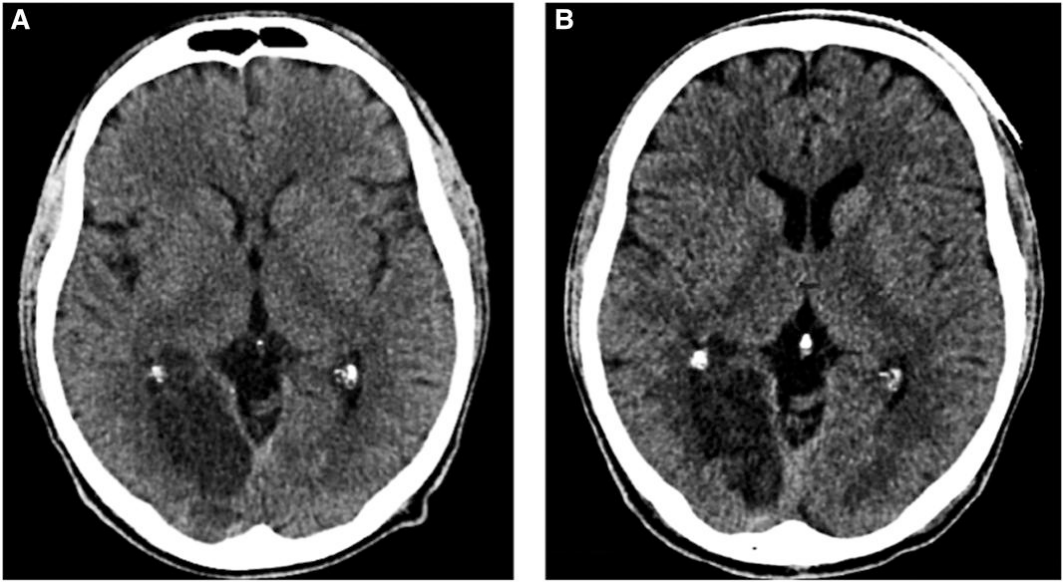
Brain CT scan without contrast performed in the emergency department (*A*) and 12 h after PCI (*B*). No bleeding transformation of the ischaemic stroke was assessed during the cangrelor infusion.
